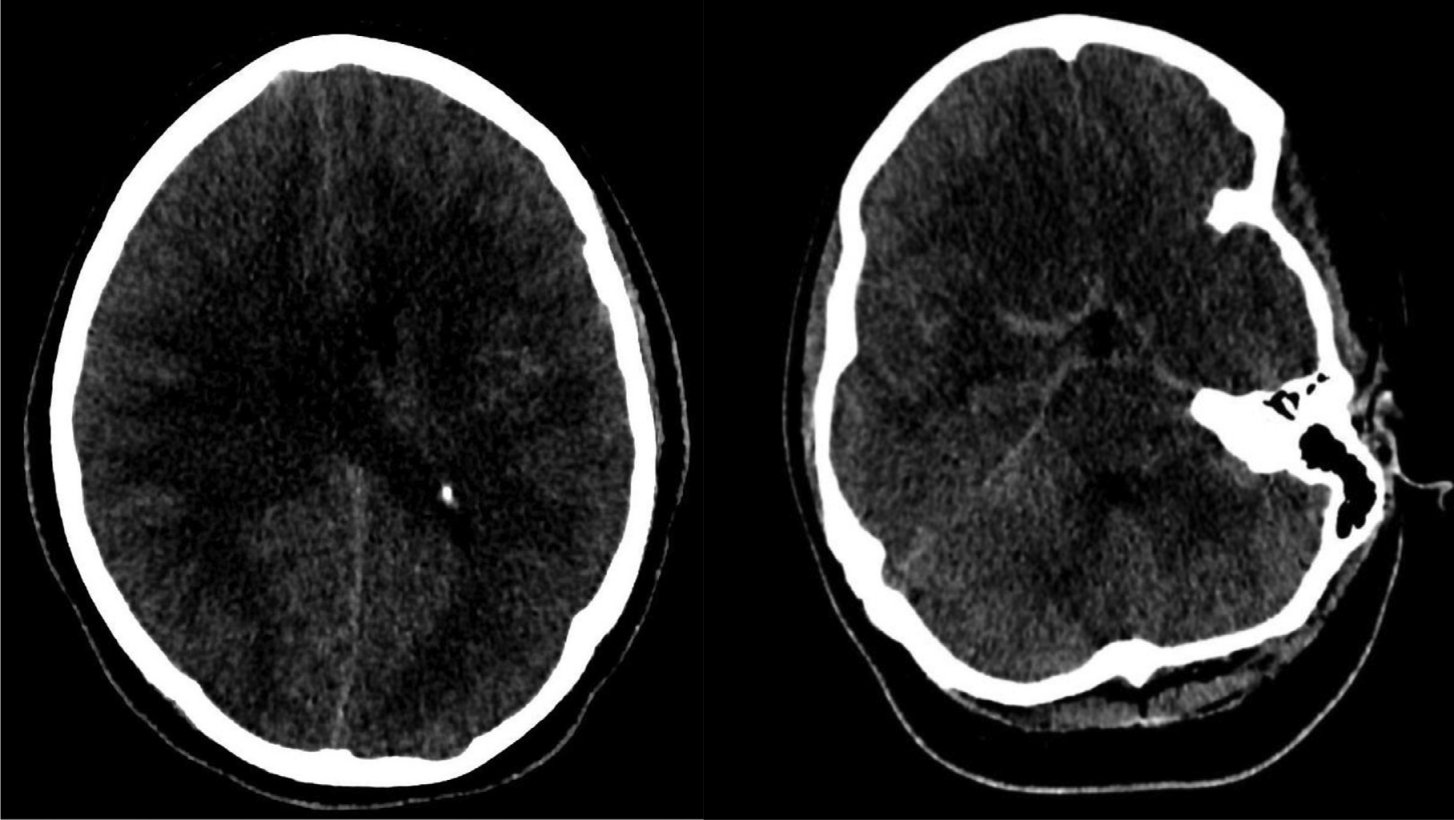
Axial non-contrast CT head images demonstrating areas of subarachnoid hyperdensities termed as pseudosubarachnoid appearance due to vascular engorgement secondary to diffuse cerebral edema and underlying history of rescusitation from cardiopulmonary arrest.
